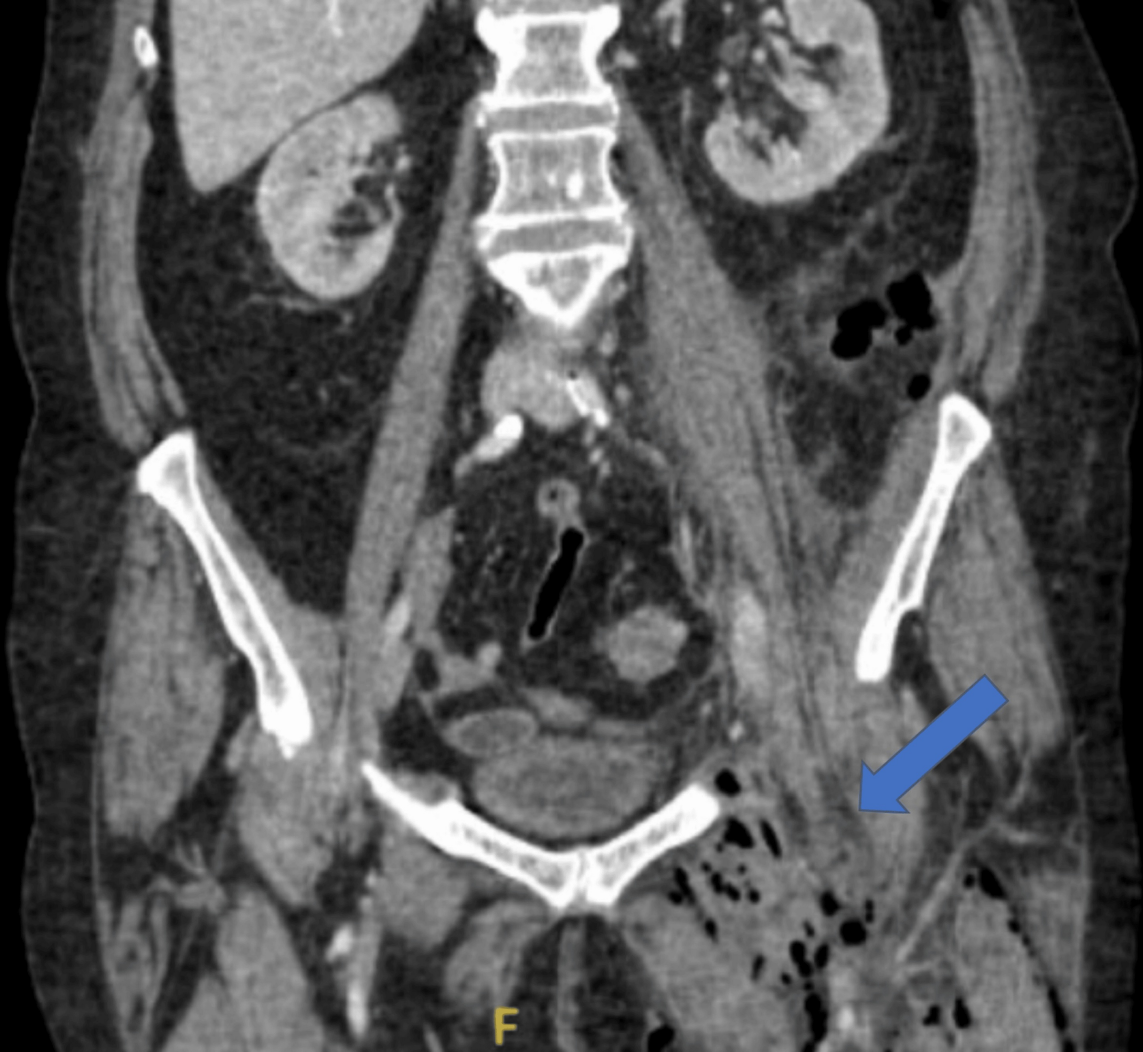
Presenting CT showing retroperitoneal gas extending into the proximal thigh along fascial planes The blue arrow highlights retroperitoneal gas.
